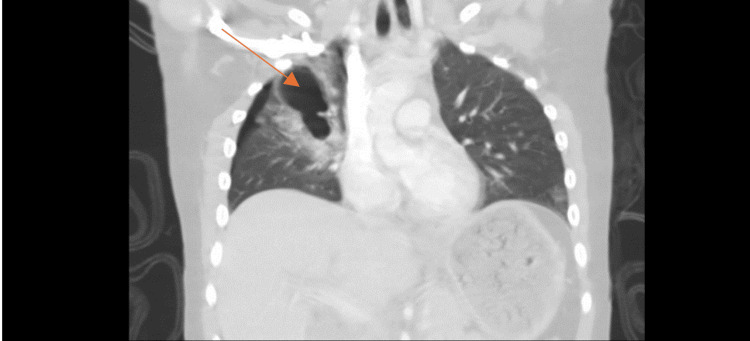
Coronal section CT of the thorax. Right-sided pneumothorax and patchy inhomogeneous airspace opacification in the right lung and multiple cavities with the air-fluid level. The arrow pointing to the cavity and opacification.
